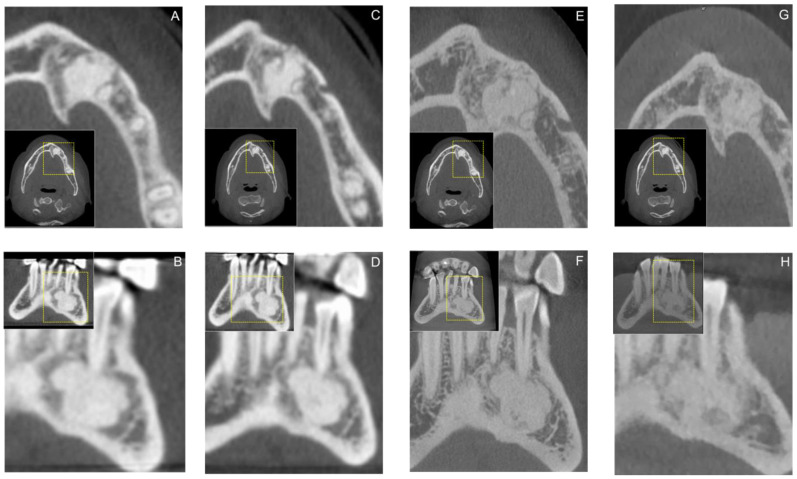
Axial (A,C,E) and Coronal (B,D,F) reconstructions of a study participants cone-beam computed tomography (CBCT) demonstrating annual radiological follow-up of cemento-osseous dysplasia. Additionally, the most recent follow-up on axial (G) and coronal (H) CBCT reconstructions of the region of interest using a low-dose imaging protocol is shown. For orientation, the dotted rectangles in the corner show the enlarged area of the region of interest.
